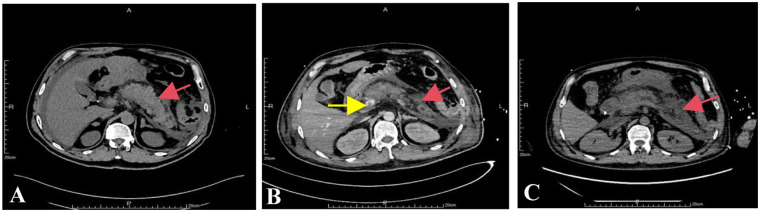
CT examination results of the patient's abdomen. Note: (A) CT examination performed in the outpatient clinic; (B) whole-abdominal enhanced CT during ICU stay; (C) Abdominal CT examination of the second review during ICU stay. The red arrow indicates the gradually expanding necrotic exudation area of the pancreas, and the yellow arrow indicates portal venous occlusion.
